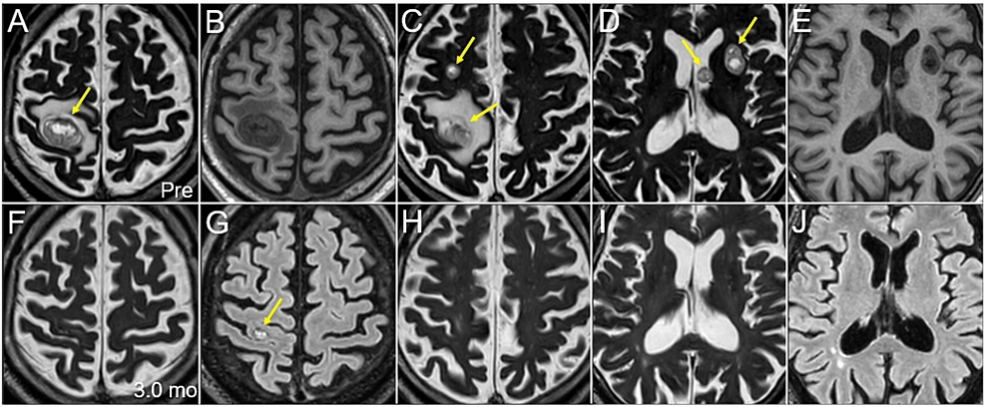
Case 1: Magnetic resonance images before and after five-fraction stereotactic radiosurgery The images show axial magnetic resonance images (MRI) (A-J); before SRS (Pre) (A-E); 3.0 months (mo) after SRS (F-J); T2-WI (A, C, D, F, H, I); T1-WI (B, E); and fluid-attenuated inversion recovery (FLAIR) images (G, J). (A-J) All images are shown in the same magnification and coordinates under co-registration and fusion. (A, C, D) A total of four mass lesions is observed (arrows in A, C, D). The intraventricular lesion probably originates from the choroid plexus in the lateral ventricle. (F-J) At three months after SRS, nearly complete remissions on T2/FLAIR images are observed in all the lesions, except for one in the right pre-central gyrus, the tumor remnant of which is only visible on the FLAIR image (arrow in G). WI: weighted image; SRS: stereotactic radiosurgery
